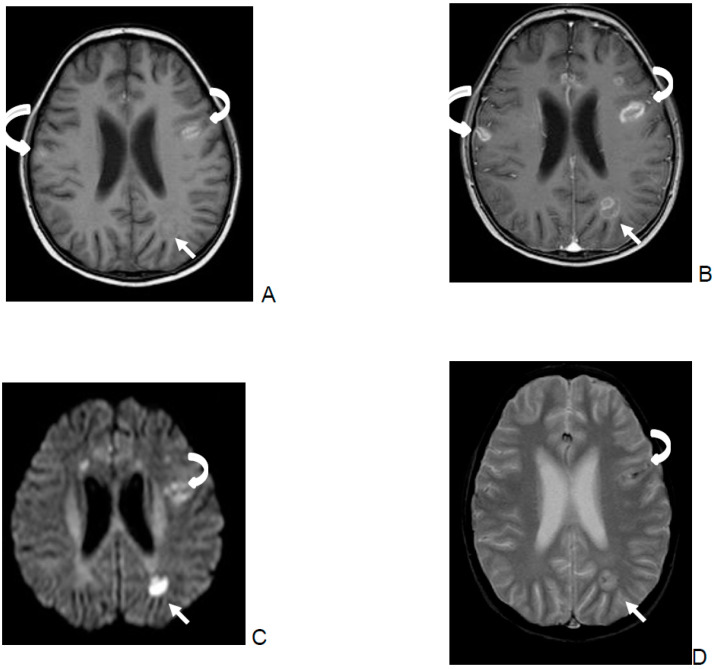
Multiple foci of hemorrhage with cerebritis and abscess formation in a 10-year-old boy with acute lymphoblastic leukemia. The patient died due to the fungal infection. The figure demonstrates the difficulties in differentiating between abscesses and parenchymal bleeding (curved arrows). 1. T1-W without contrast (A) reveals multiple hyperintense foci of hemorrhage (curved arrows) with gadolinium enhancement of magnetic resonance imaging (MRI) lesions on T1-W image after contrast (B). Enhancement in areas of parenchymal bleeding may have different patterns, such as ring, focal, and diffuse–patchy. Because of the hemorrhagic nature of the lesions, a hypointense rim or foci are usually present in SWI or GRE T2* (D). Factors such as oxyhemoglobin in the hyperacute stage or extracellular methemoglobin in the late sub-acute stage affect how the DWI (C) signal appears in the presence of hemorrhage. These substances are thought to give parenchymal hemorrhage the hyperintense appearance on DWI (curved arrow, C) and low ADC values, which is similar, but usually not as strong as the restricted diffusion seen in an abscess (straight arrow, C). 2. The abscess (white straight arrow) shows a small hyperintensity in T1-W without contrast (A), clear restricted diffusion within the core of the lesion (straight arrow, C) with a ring like enhancing lesion (B) and hypointense foci in GRE T2* (D). Brain abscesses due to a fungus are usually hypointense on the T1-W image before contrast, but show small areas of hyperintensity due to the presence of iron, manganese, or methemoglobin (A).
